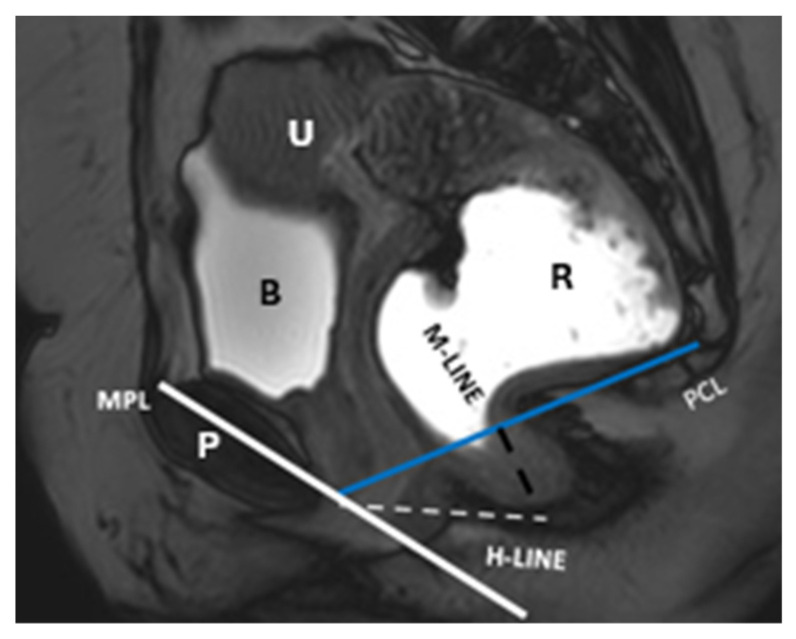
Sagittal T2-weighted MR defecography at squeeze shows normal pelvic anatomy. The pubococcyxgeal line (PCL), H line, M line, and mid-pubic line (MPL) are displayed. The anorectal junction aligns with the PCL, the H and M lines measure within normal limits, and no pelvic floor descent or organ prolapse is observed. Identifiable structures include the pubic symphysis (P), rectum (R), bladder (B), and uterus (U).
